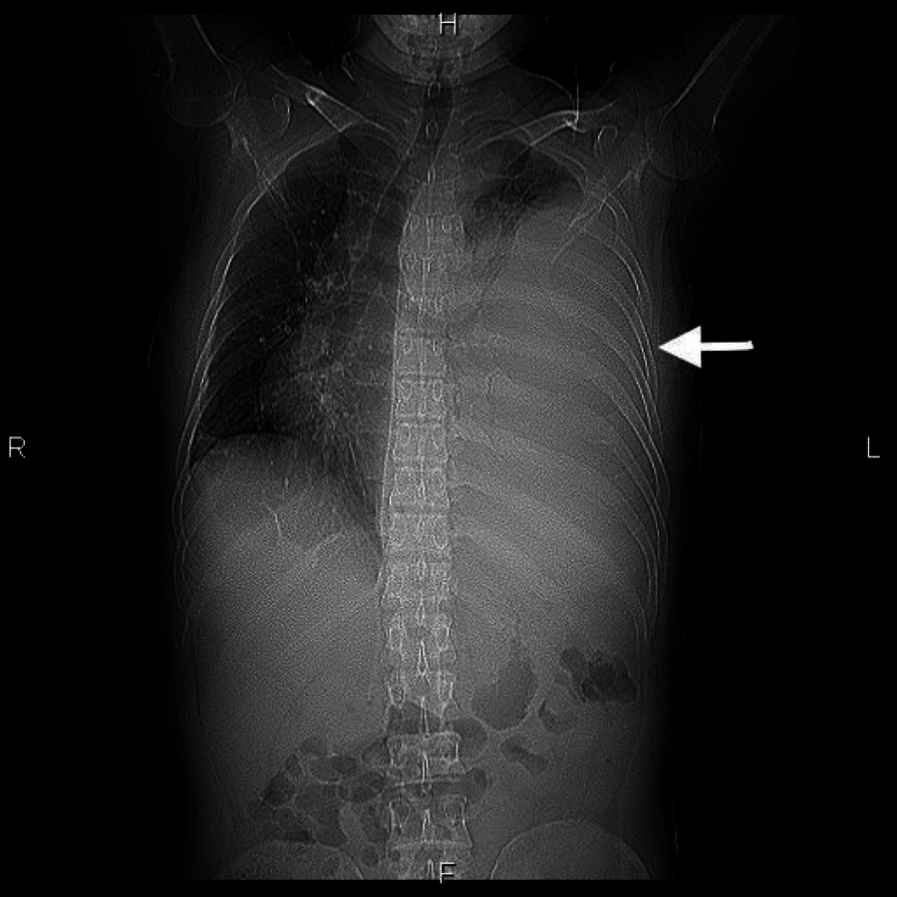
Chest X-ray showing a large left-sided pleural effusion
